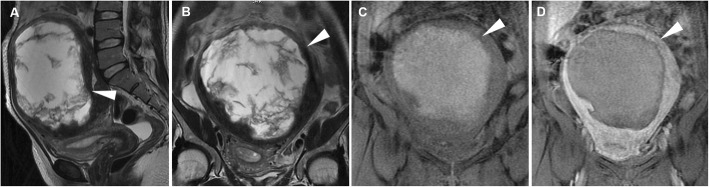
Leiomyoma with haemorrhagic degeneration in a 37-year-old woman with a palpable abdominal mass, pelvic pain and fever. Sagittal (a) and oblique-coronal (b) T2-weighted images show a huge subserosal leiomyoma arising from the posterior wall of the uterus, with heterogeneous signal intensity (arrowheads). On oblique-coronal fat-suppressed T1-weighted image (c), the mass demonstrates hyperintense signal (arrowhead) consistent with haemorrhagic degeneration, without contrast enhancement after gadolinium administration (d)
